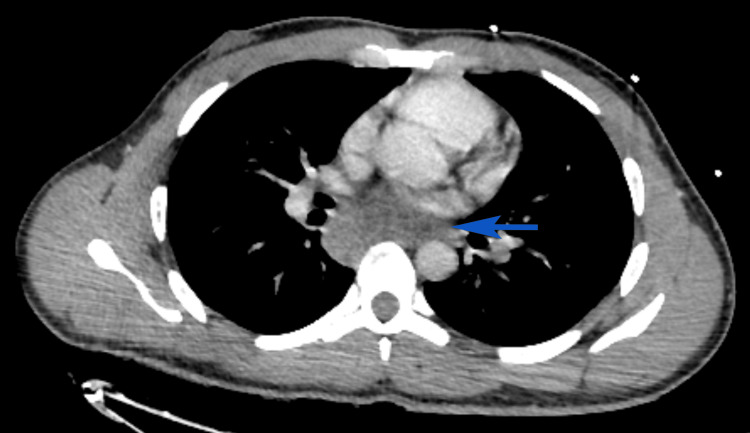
CT thorax axial view demonstrating a heterogeneous, septated posterior mediastinal mass measuring 31 × 66 mm in axial dimensions and 61 mm in craniocaudal length. CT: computed tomography, blue solid arrow: heterogeneous posterior mediastinal mass
